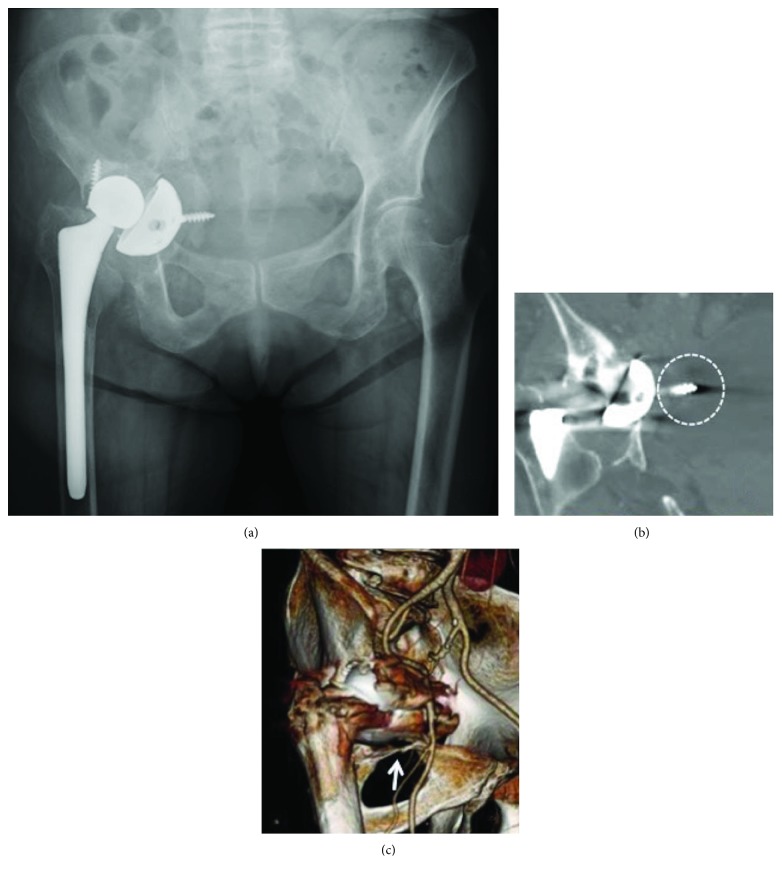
Preoperative plain radiographic (a) and computed tomographic (b, c) images of the pelvis. Coronal computed tomographic image shows a screw pressing the bladder wall (white dotted circle). White arrow indicates a fracture of the superior aspect of the obturator foramen.
